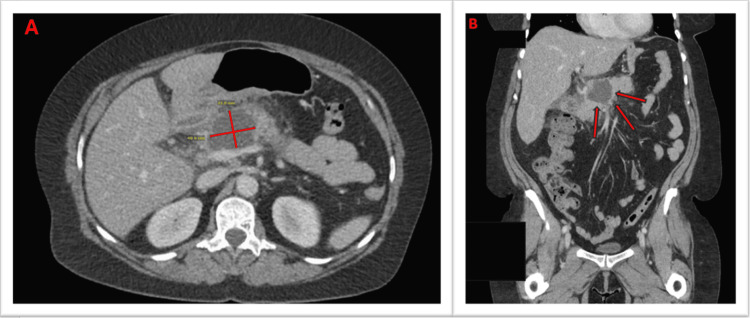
Axial and coronal view contrast-enhanced CT scan of the abdomen Contrast-enhanced CT scan of the abdomen depicts mesenteric stranding with a small amount of ascites. The pancreas showed a 5.1 cm × 4.1 cm hypodensity focus of the proximal aspect of the pancreas. The distal pancreas is unremarkable. Diffuse peripancreatic stranding. No dilatation of the pancreatic duct. Also noted in the peritoneum was a relatively new, irregular, lobulated, and loculated fluid collection of the right upper quadrant near the gallbladder fossa and caudate lobe. Panel A depicts an axial view, while panel B depicts a coronal view.
